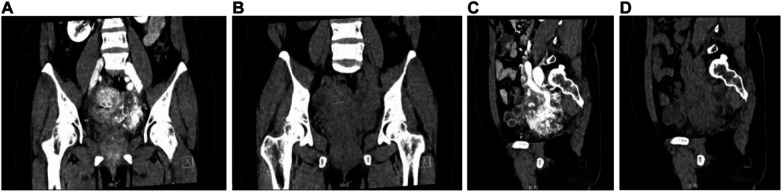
Preoperative pelvic enhanced computed tomography. (A) Pelvic coronal reconstruction section (uterus overall morphology + fibroids); (B) Pelvic coronal reconstruction section (right adnexal area); (C) Pelvic contrast-enhancement coronal section (vascular lesions on the left side of the pelvis); (D) Pelvic contrast-enhanced coronal section (leiomyoma enhancement manifestation).
